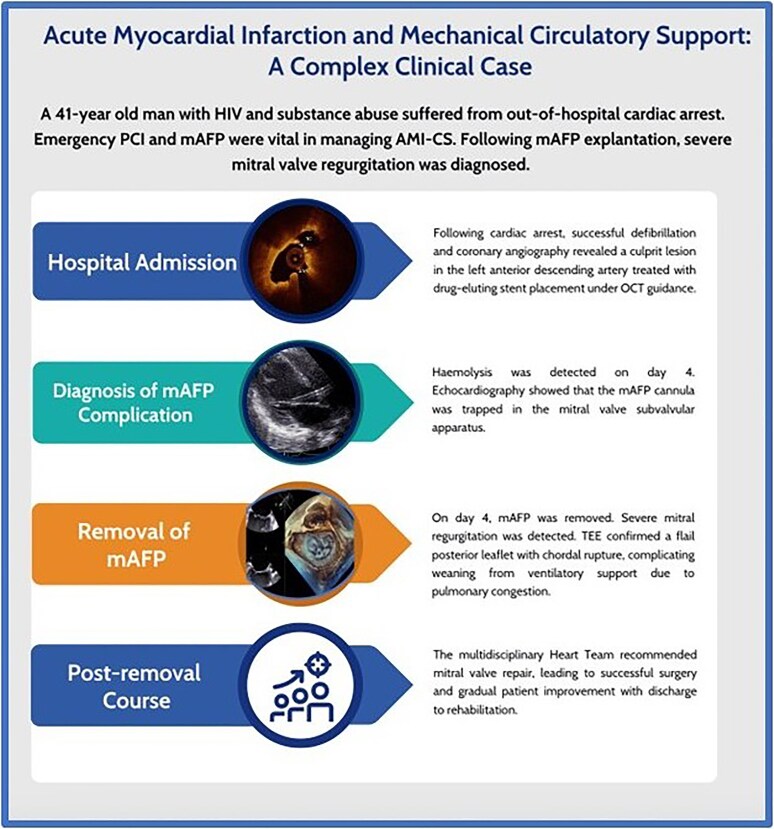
Timeline summary of acute myocardial infarction and microaxial flow pump-associated mitral valve complication in a critically ill patient. The infographic summarizes the sequence of clinical events in a 41-year-old man with HIV and substance abuse who suffered an out-of-hospital cardiac arrest. Following successful defibrillation and coronary revascularization with OCT-guided stent implantation, a mAFP was inserted for haemodynamic support due to cardiogenic shock. On Day 4, haemolysis was detected and echocardiography revealed entrapment of the mAFP within the mitral valve subvalvular apparatus. The device was subsequently removed and TEE confirmed severe mitral regurgitation caused by a flail posterior leaflet with chordal rupture. The multidisciplinary Heart Team recommended early surgical intervention. Mitral valve repair was successfully performed, allowing for gradual clinical improvement and discharge to rehabilitation. Abbreviations: AMI-CS, acute myocardial infarction-related cardiogenic shock; HIV, human immunodeficiency virus; mAFP, microaxial flow pump; OCT, optical coherence tomography; PCI, percutaneous coronary intervention; TEE, transoesophageal echocardiography.
